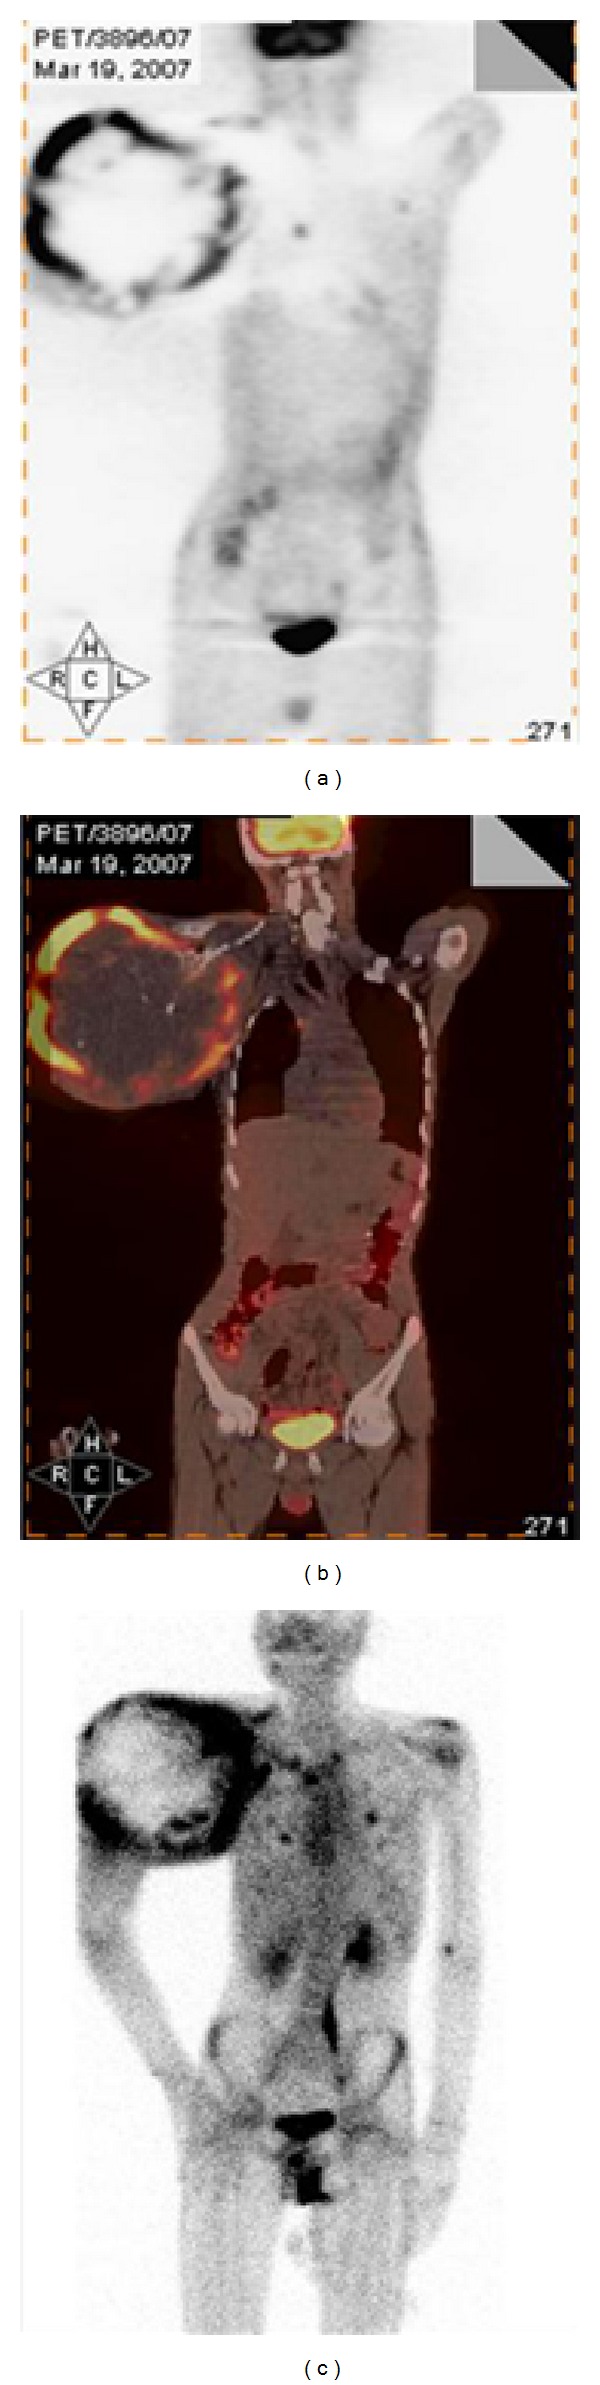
Showing intense [^18^F]FDG uptake (a) and the ^99m^Tc-DMSA (V) uptake (c) at the primary site of Rt humerus.
